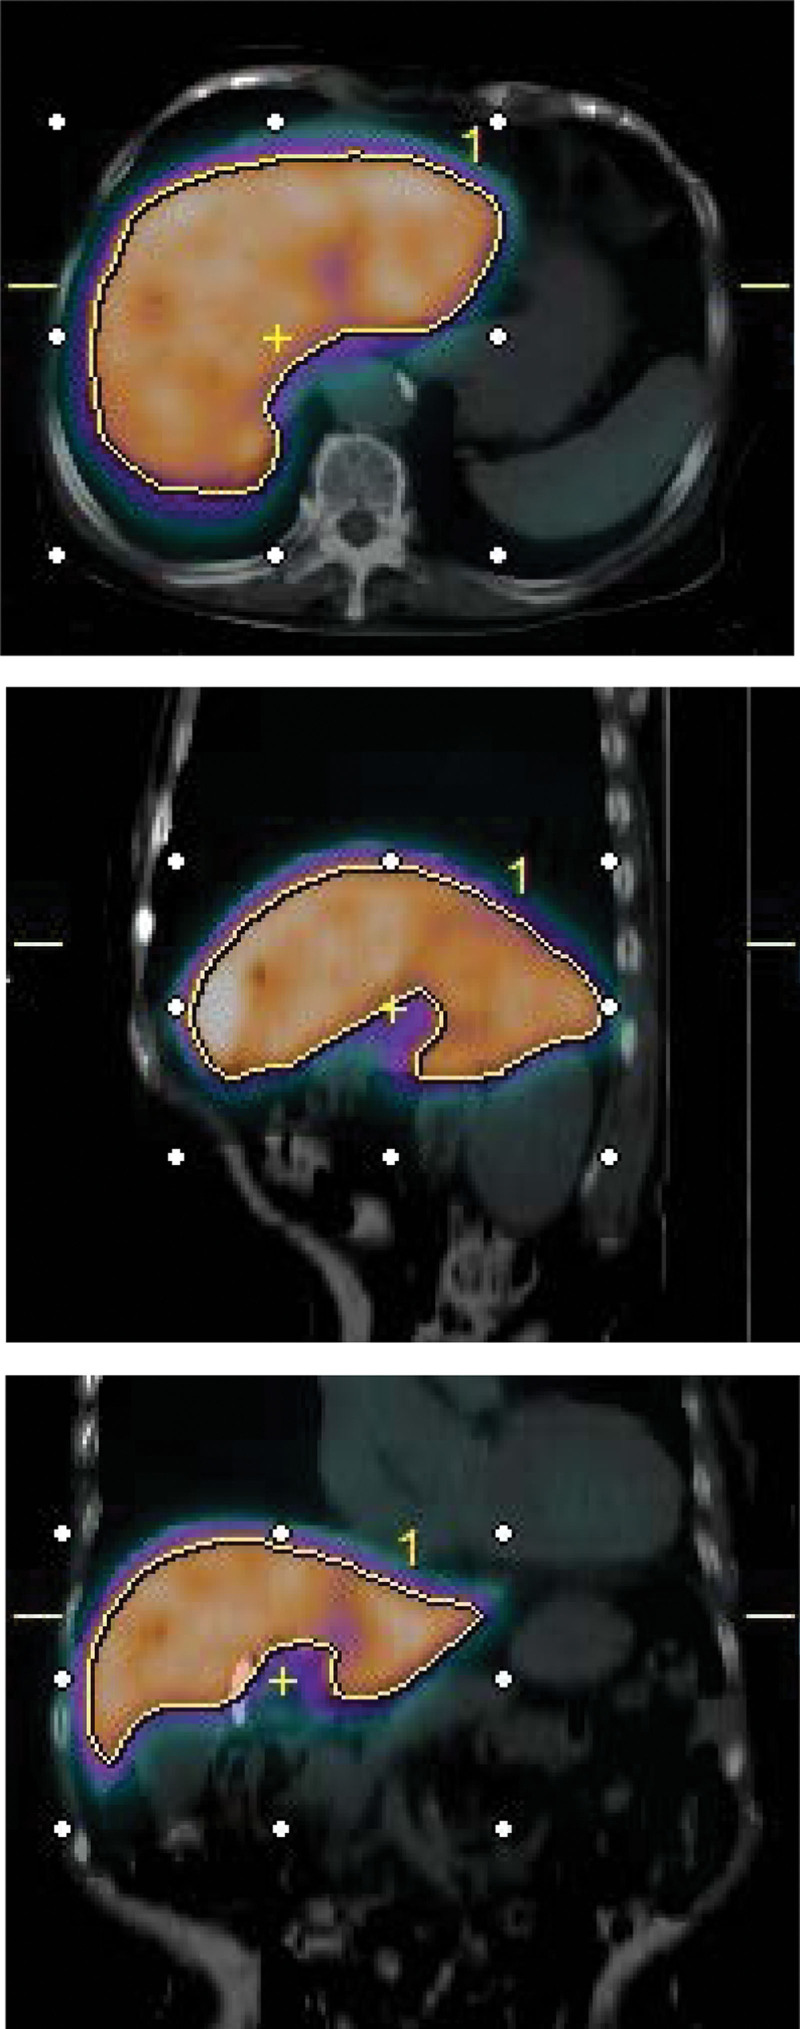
To measure the mean standardized uptake value (SUV_mean_), a region-of interest (ROI) was established over the entire liver on single-photon emission-computed tomography (SPECT-CT) fusion image. All galactosyl human serum albumin (GSA) SPECT images were acquired 20 minutes after intravenous Tc-99m-diethylenetriamine-penta-acetic acid (^99m^Tc)-GSA injection. ROI setting was performed using GI-BONE; GI-BONE is an application for SUV calculation that automatically sets the threshold value for ROI. The threshold value was set to SUV 3. ROI = region-of interest, SPECT-CT = single-photon emission-computed tomography. SUV = standardized uptake value.
